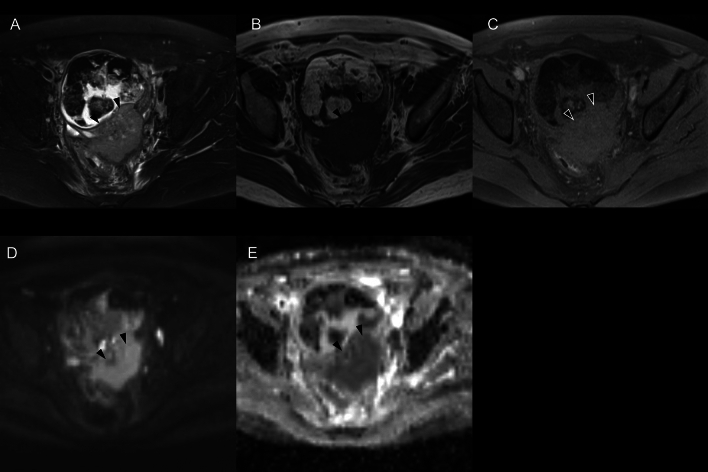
Squamous cell carcinoma arising in ovarian mature cystic teratoma with transmural extension in a 53-year-old woman. a Axial fat-suppressed T2-weighted image (T2WI) shows a cystic lesion in the pelvis with a solid component (arrowhead). The solid component protrudes from the wall of the cyst toward the dorsal side, suggesting transmural extension and does not have any fat signal or calcification. b, c Axial T1-weighted image (T1WI) and fat-suppressed T1WI reveal hyperintensity in the cystic component on T1WI, which changes to hypointensity on fat-suppressed T1WI, confirming the presence of fat. d, e The solid component shows hyperintensity on diffusion-weighted images and is hypointense on apparent diffusion coefficient maps
